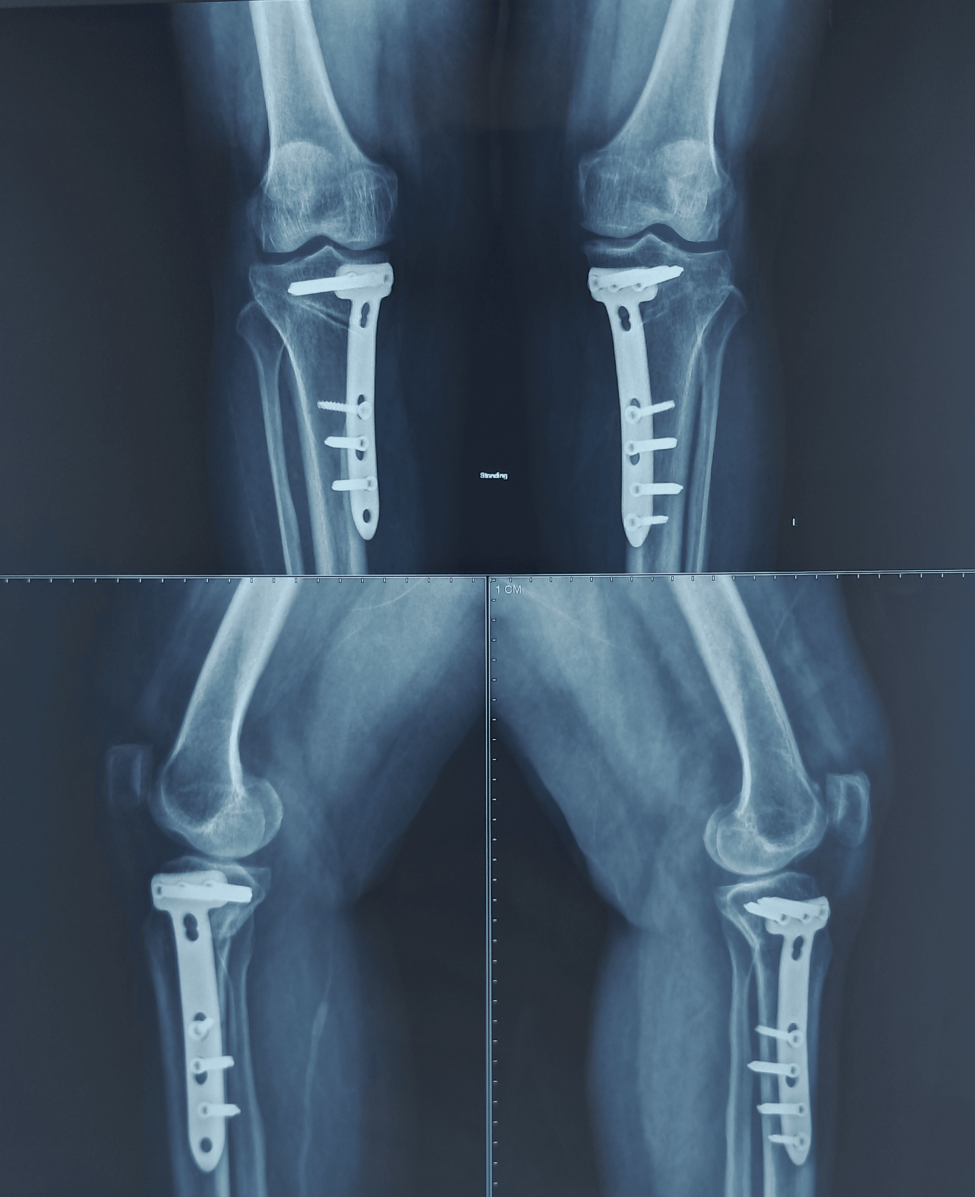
Anteroposterior and lateral radiographs at one year of follow-up showing union of all the cortices. The fracture line is visible only in the AP view of the right knee.
